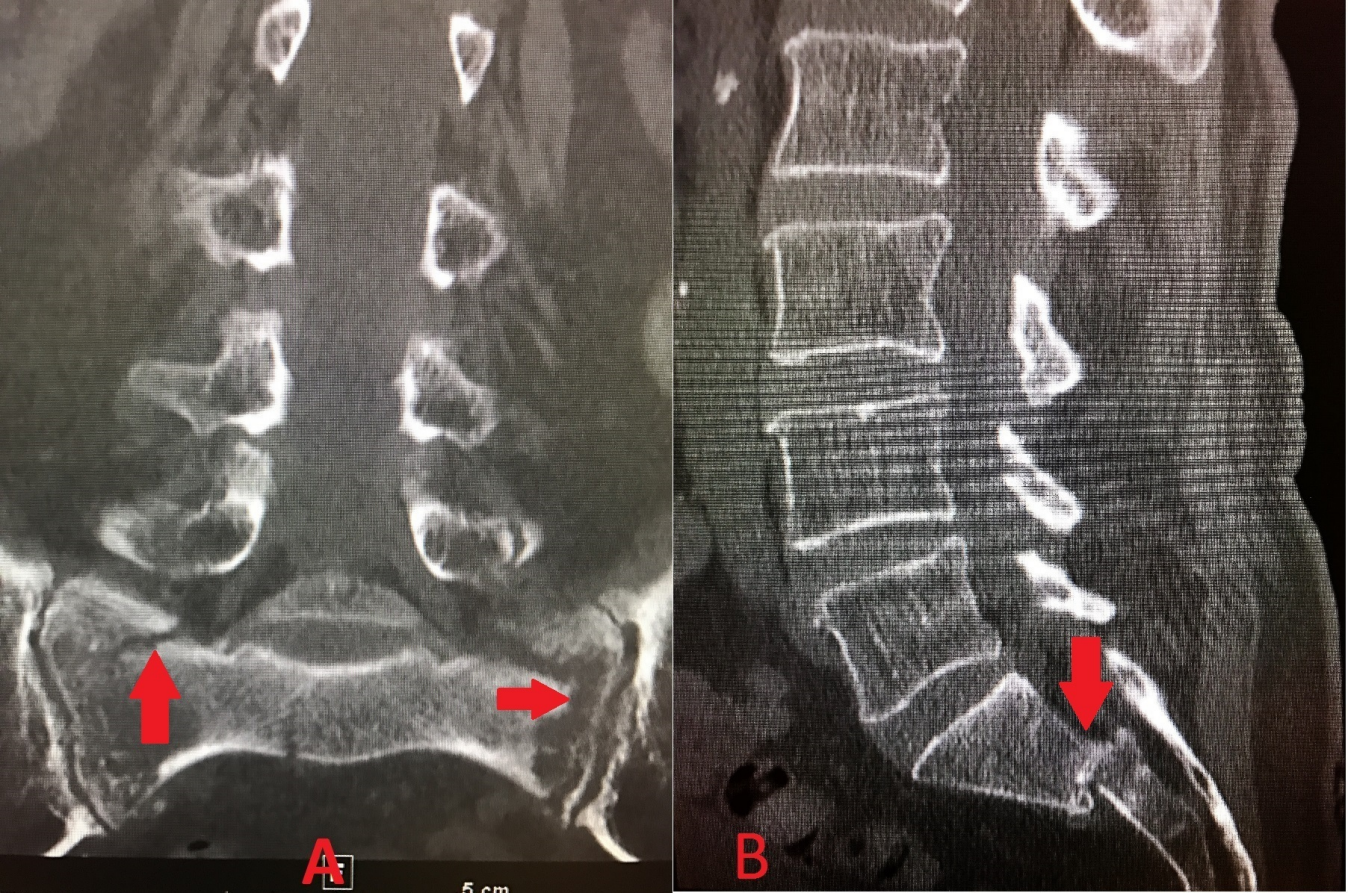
CT of the lumbar spine, sacrum, and pelvis A. Coronal view showing comminuted SIFs (arrows) through the bilateral sacral ala; B. Sagittal view showing S1-S2 anterolisthesis (arrow)
